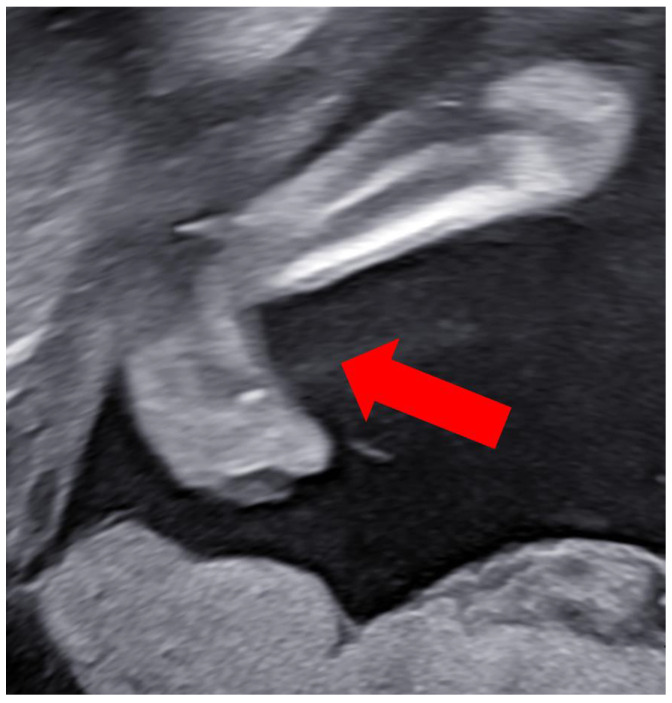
Clubfoot on the left side. Transabdominal ultrasound.
